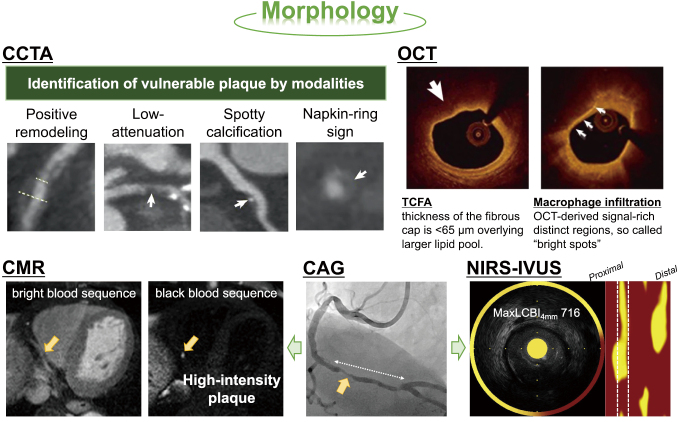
Morphological findings of vulnerable plaques on coronary modalities The findings of vulnerable coronary plaque identified by both invasive and non-invasive modalities, such as CCTA, non-contrast CMR, OCT, and NIRS-IVUS, are shown. The upper panel shows the representative vulnerable characteristics detected by CCTA. The upper right panel shows the TCFA and macrophage filtration using OCT. The lower panel shows that CMR-derived high intensity plaque, IVUS-derived attenuated plaque, and NIRS-derived large lipid-rich plaque defines as maxLCBI4mm ≥400 are measured in culprit lesion site of segment 3 indicated by the yellow arrow on CAG imaging. CAG, coronary angiography; CCTA, coronary computed tomography angiography; CMR, cardiac magnetic resonance; MaxLCBI4mm, maximum lipid core burden index within any 4-mm long segment; NIRS-IVUS, near-infrared spectroscopy and intravascular ultrasound; OCT, optical coherence tomography, TCFA, thin-cap fibroatheroma. Reorganized from the references of 17) and 19) with permission from the publisher.
